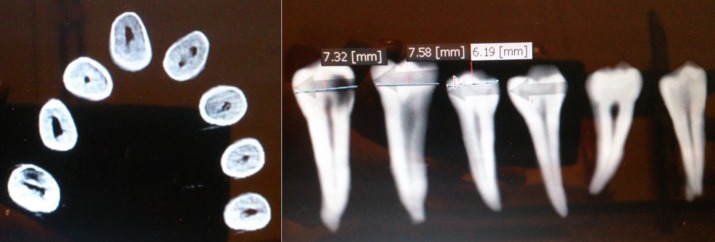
Coronal and axial sections of CBCT images of subjects
